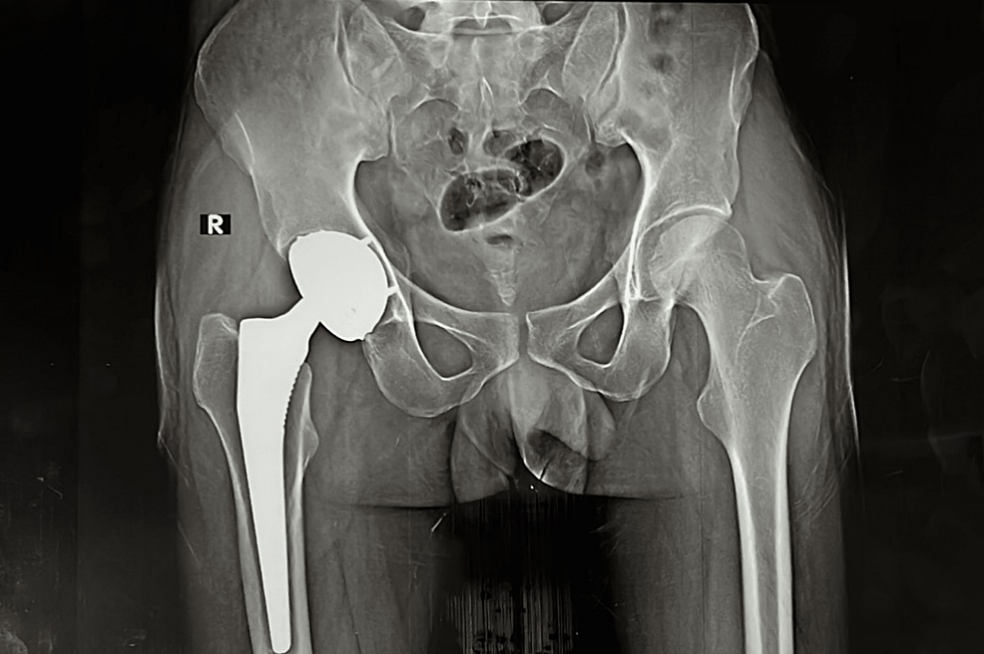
Sixteen weeks postoperative X-ray
